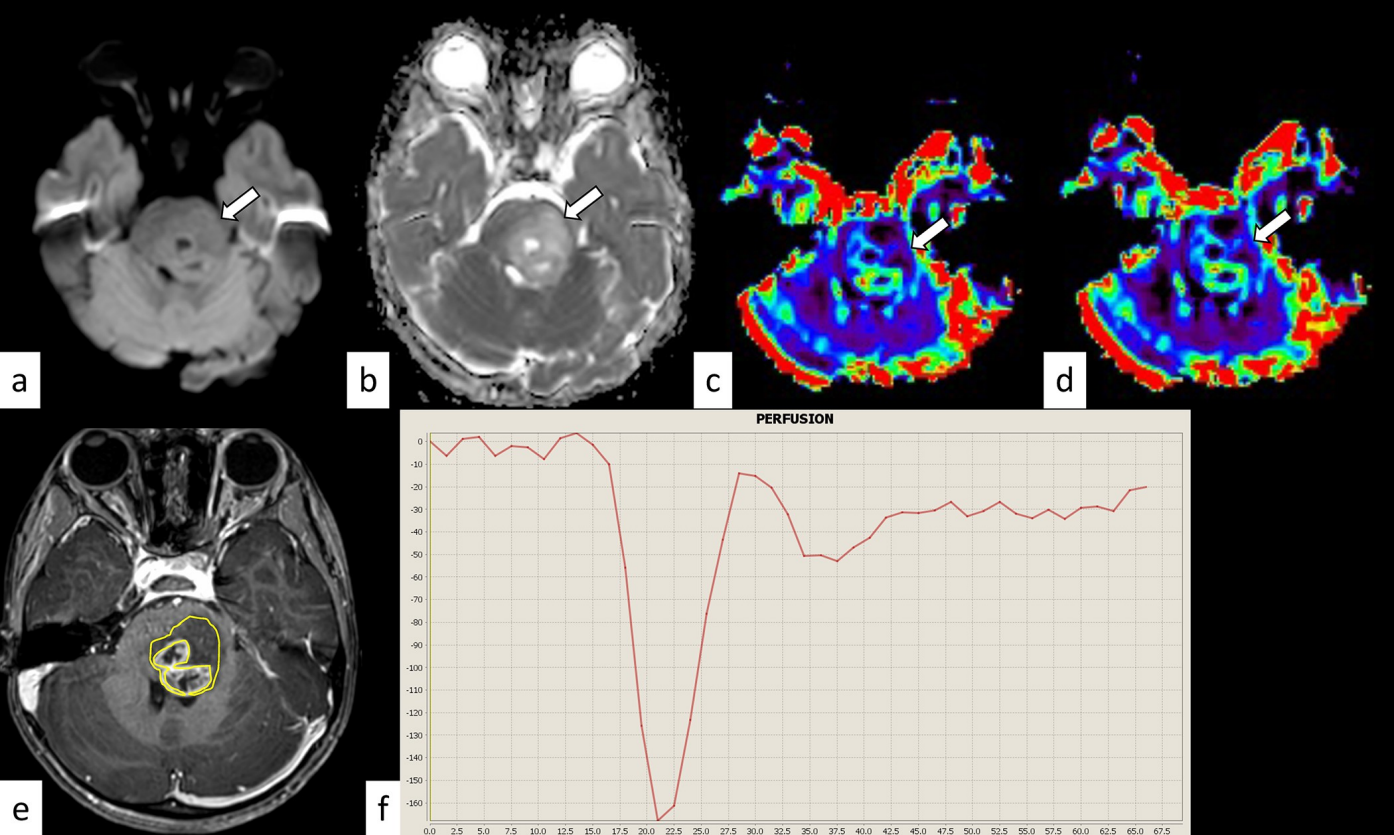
H3.1 variant diffuse midline glioma, H3K27-altered in a 4-year-old girl. The mass shows isointensity on diffusion-weighted imaging (a, arrow) and an increased apparent diffusion coefficient (nADCmean = 1.72) (b, arrow). The normalized relative cerebral blood volume and flow are 1.76 and 1.56, respectively (c and d, arrows). The corresponding post-contrast T1-weighted image and region-of-interest in this slice are shown (e). The percentage signal recovery of the mass is 0.78 (f). nADCmean = normalized mean apparent diffusion coefficient.
